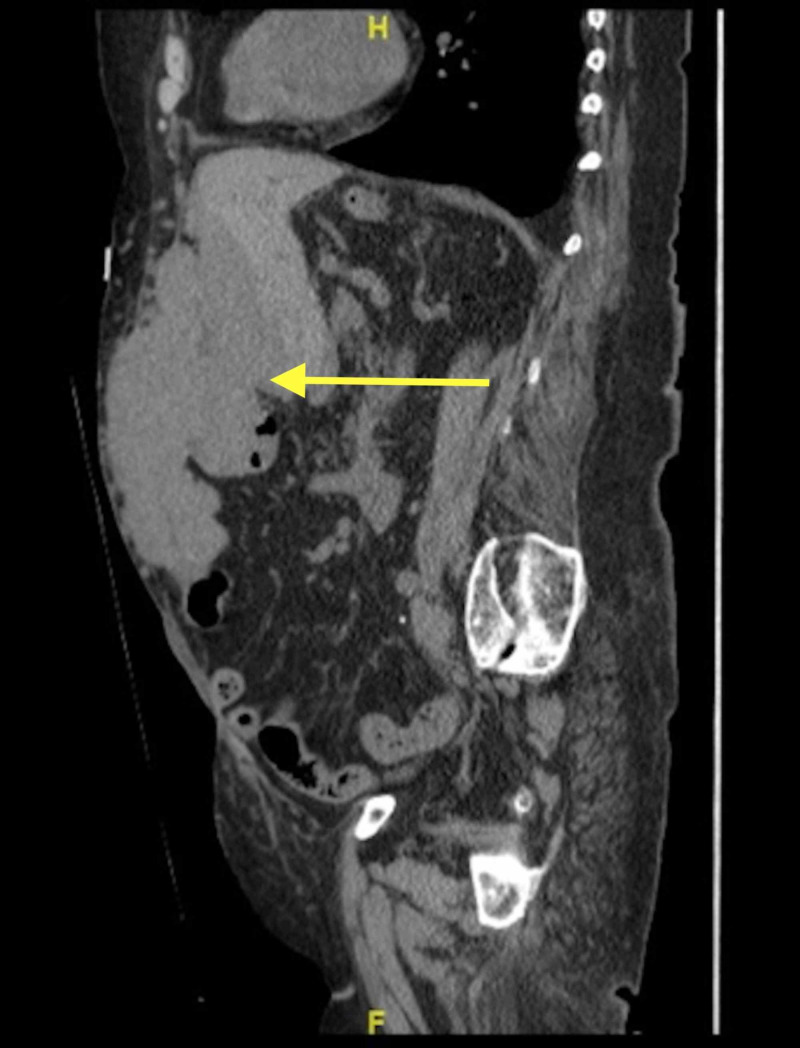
Sagittal CT with IV contrast showing a soft tissue abutting anterior liver, and infiltrating anterior abdominal wall.
